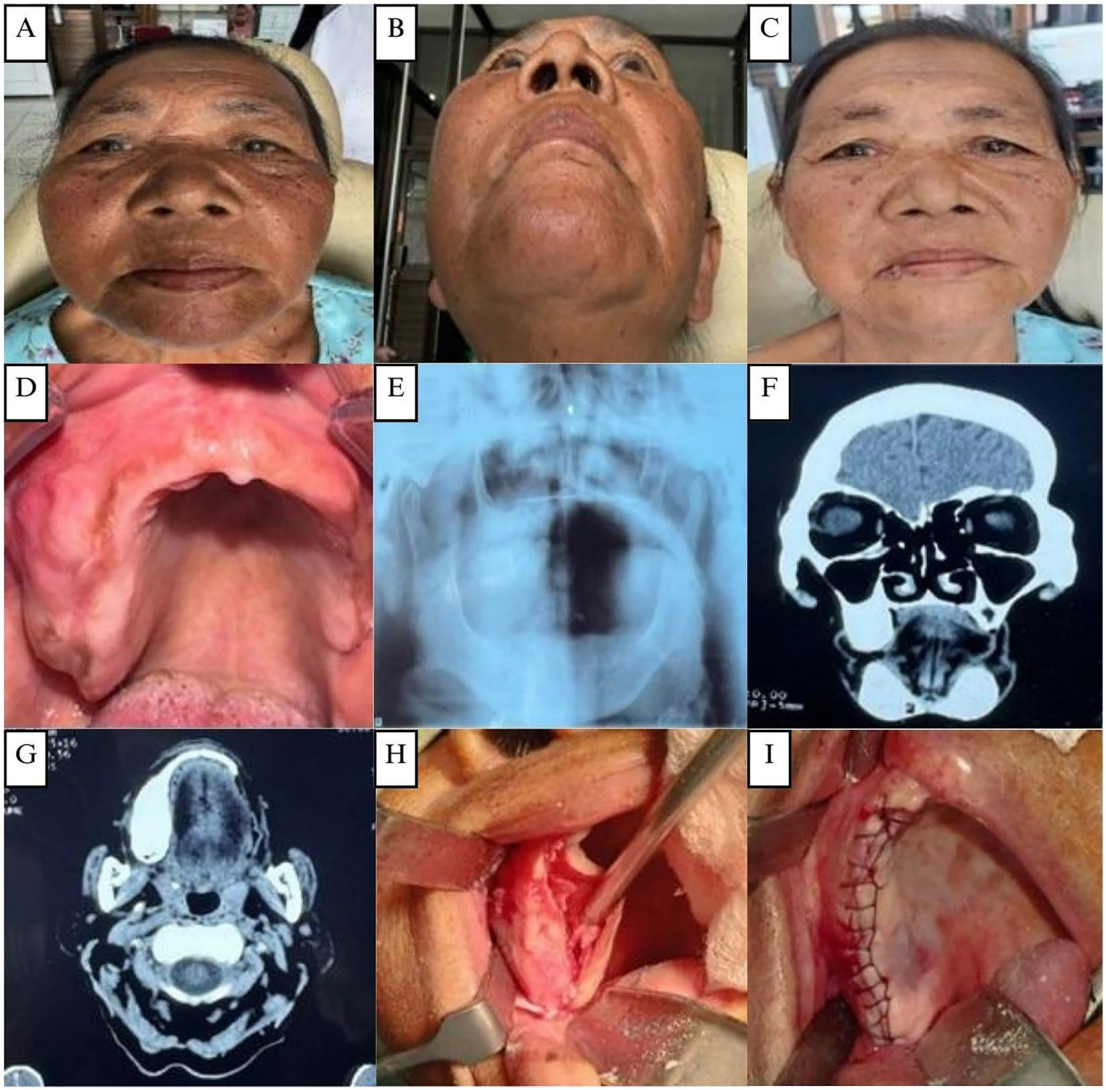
(A and B) The first case, a 62-year-old elderly female patient with swelling in the right maxillary area. (C) Clinical appearance 2 weeks post-operative. (D) Pre-operative intraoral clinical appearance (E). OPG showing a fully edentulous area with no osteodestruction. (F–G) CT scan showing a hyperdense lesion in the right maxilla measuring 28x14 mm. (H–I) Surgical exposure, bone reshaping, and layers of closure of the right maxillary alveolar arch.
